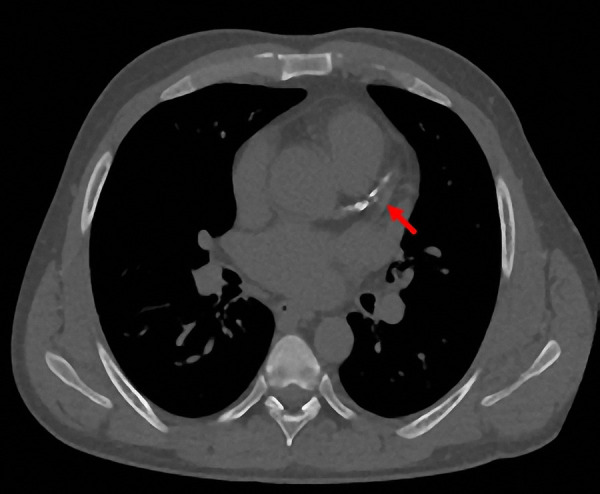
Computed tomography showing a small curvilinear metallic density at the right ventricular apex (red arrow).
